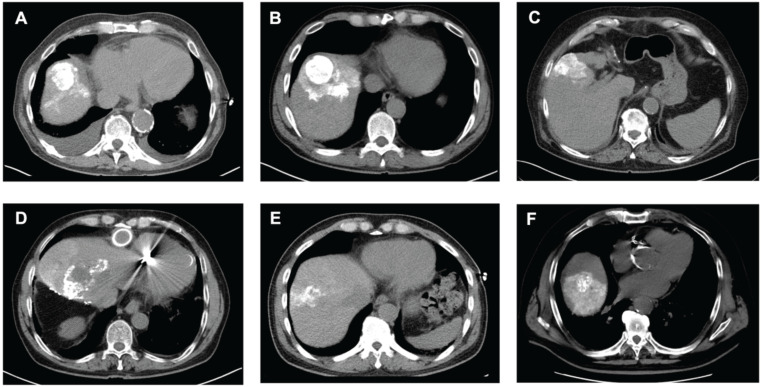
Analysis of Lipiodol Deposition and Distribution Patterns post-cTACE. Legend: CT scans obtained 24 hours after cTACE show different patterns of Lipiodol deposition within target tumors. Homogeneous deposition (A-C) was defined as ≥85% of the tumor volume containing medium or high-density Lipiodol. Heterogeneous deposition (D-F) was defined as <85% intratumoral Lipiodol uptake, characterized by patchy, irregular, or incomplete filling of the tumor. Lipiodol distribution was classified as confined to the tumor, without visible extension into the surrounding liver parenchyma (i.e., A), extending into one adjacent liver segment, without extension into additional segments (i.e., B, E, F), or extending beyond the tumor-bearing segment into at least one adjacent liver segment (i.e., D).
